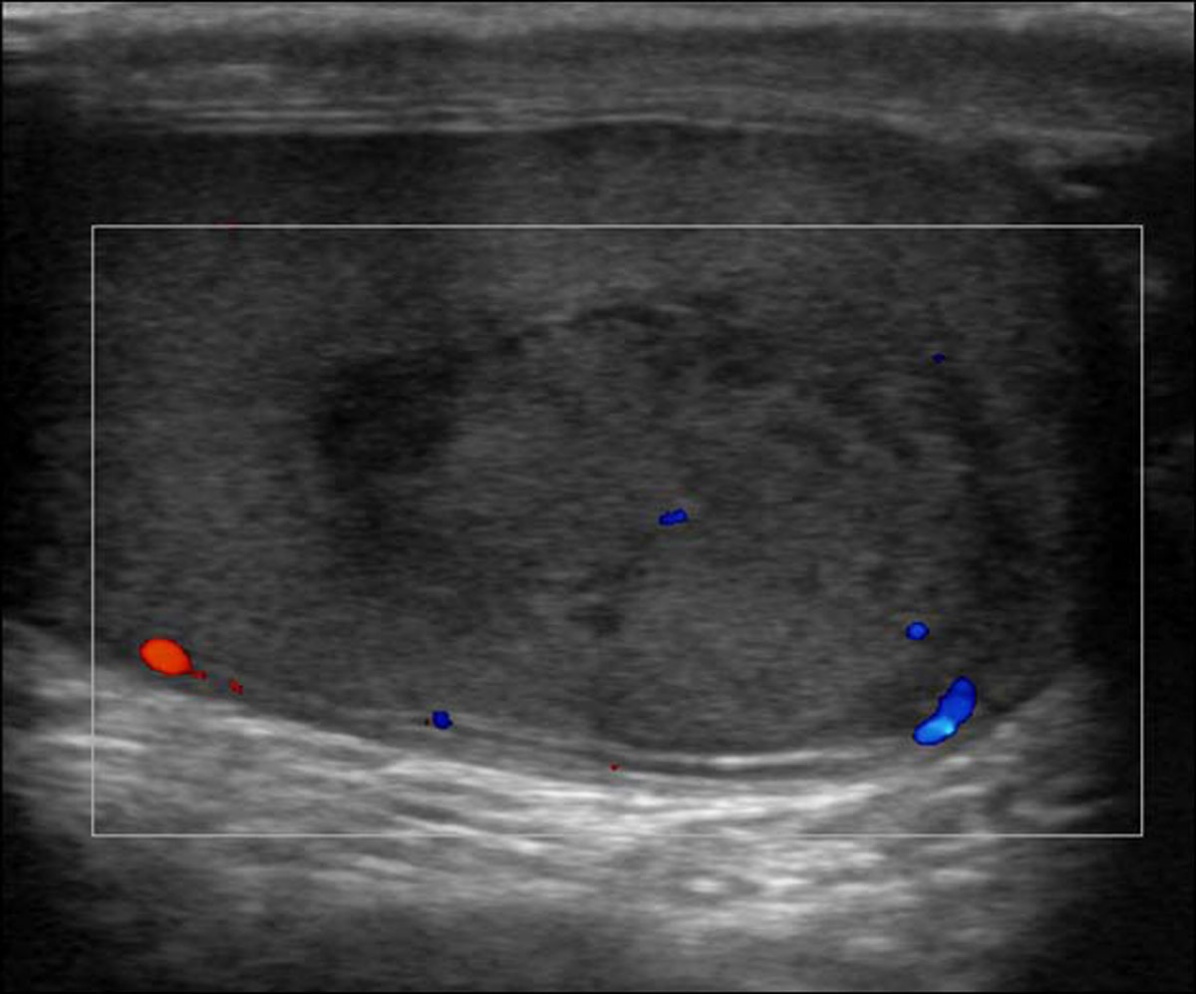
Gray scale and Colour Doppler of testicular trauma. In this type of injury, US images also demonstrate poorly defined testicular margins and heterogeneous echotexture, with focal hyperechoic or hypoechoic areas in the testicular parenchyma corresponding to areas of hemorrhage or infarction . Color and duplex Doppler images may show decreased flow or no flow.
